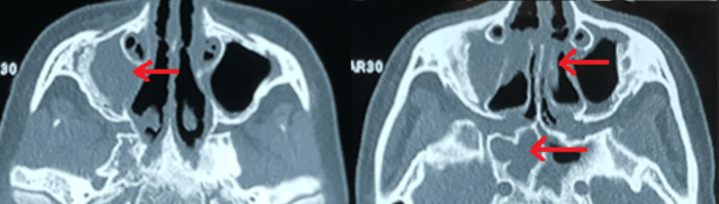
CT scan of the sinus showing filled maxillary and sphenoid sinuses with wall thickening, a polypoid mass of the right middle meatus and nasal septum erosion
